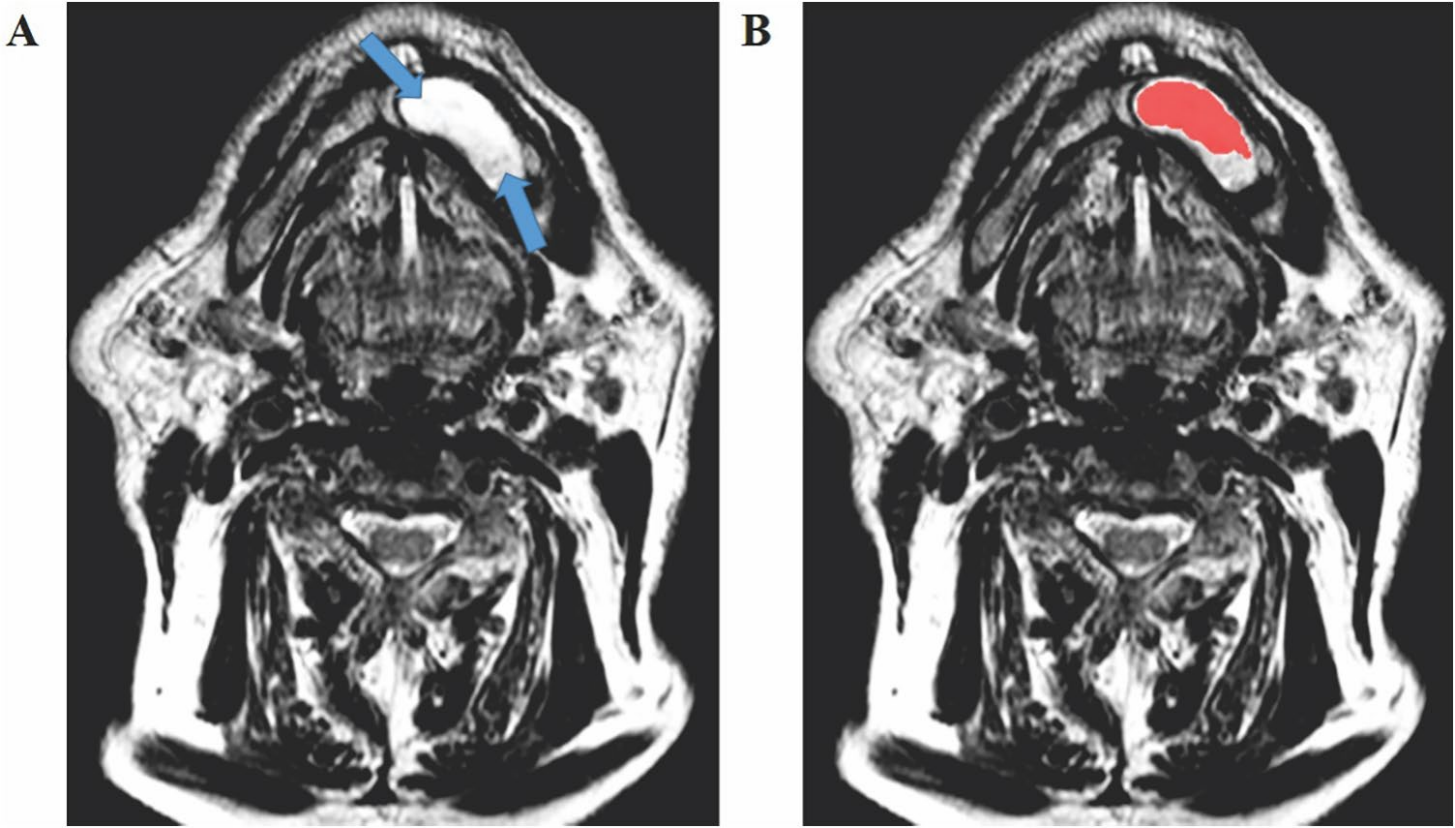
(A) Ameloblastoma with smaller volume compared to that shown in Fig. 1. However, the variable signal intensity along the entire length of the lesion is maintained, so that the most homogeneous region remains restricted to the portion characterized by the hypersignal, which is typical of T2-weighted sequences. (B) Segmentation process represented by the red contour. Only the most homogeneous portion of the lesion was segmented, that is, the region shown by the hypersignal.
